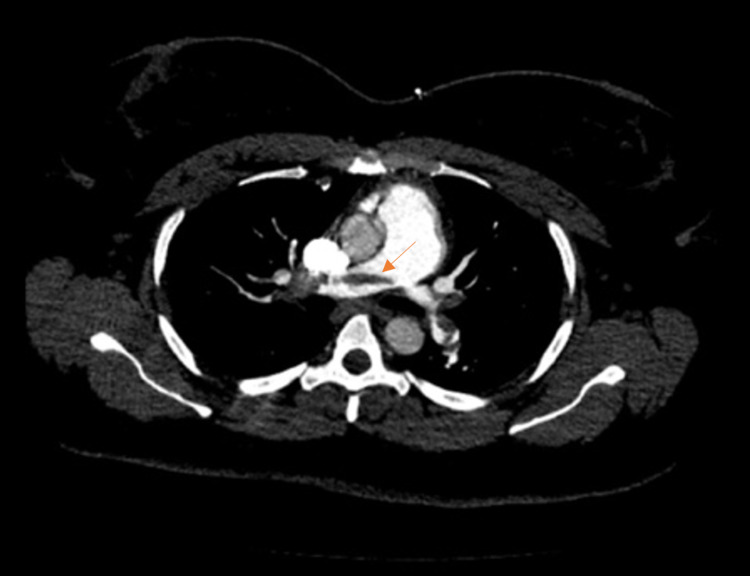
Computed tomographic angiography of the chest (axial view) Computed tomographic angiography of the chest demonstrating a large saddle embolus (orange arrow) traversing both right and left main pulmonary arteries with additional emboli distally.
